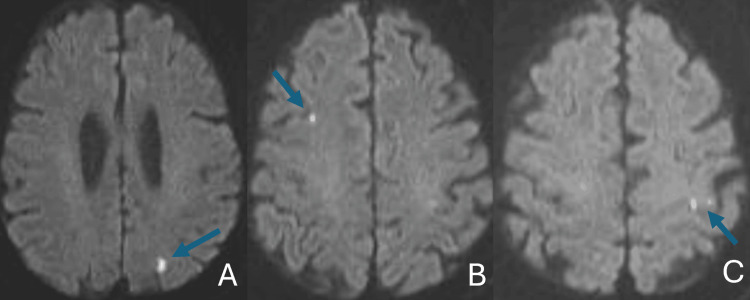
MRI of brain diffusion images (A-C) demonstrating bilateral infarcts (arrows)
